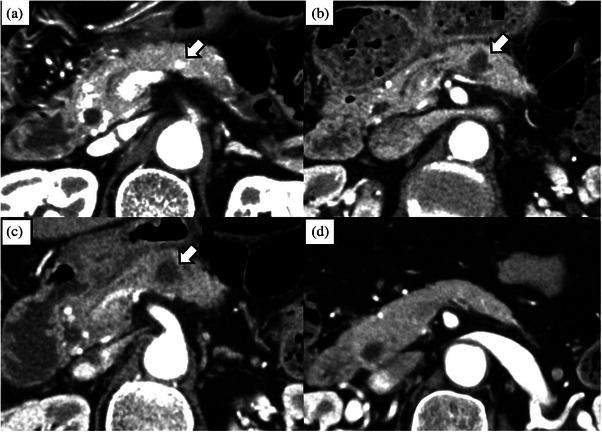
Patients without complications after EUS‐guided radiofrequency ablation (Patient number 4). (a): An enhanced tumor measuring 10 mm located in the head of the pancreas on CE‐CT (arrow). (b): CE‐CT performed 3 days after the procedure reveals that the tumor site is ablated (arrow). (c): CE‐CT at 1 month showing that the enhancement of the tumor had disappeared (arrow) and the ablated site was still present. (d): The ablation site cannot be identified on CE‐CT at 6 months. CE‐CT, contrast‐enhanced computed tomography; EUS, endoscopic ultrasonography.
